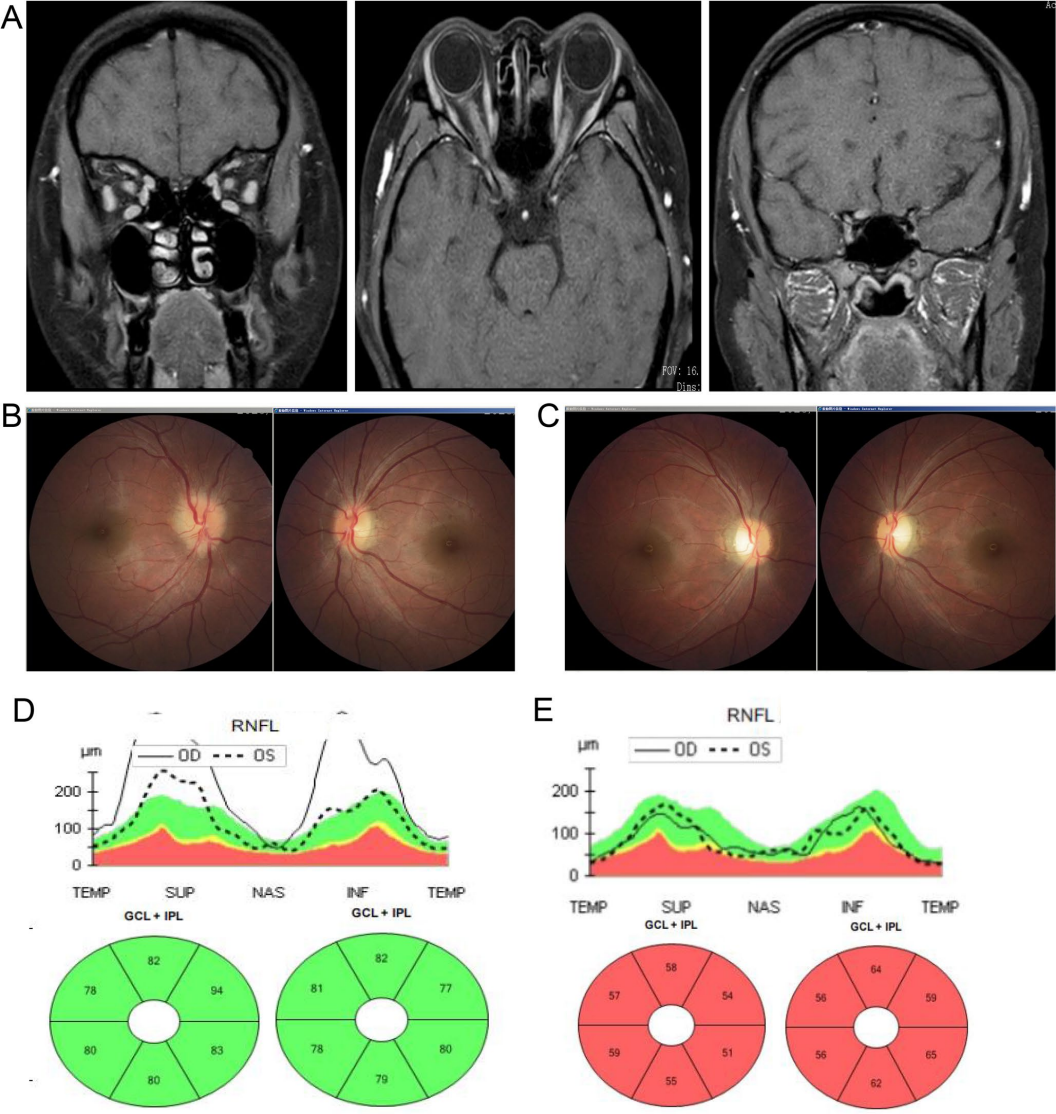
A 26-year-old woman without a significant past medical history presented with acute blurred vision of her both eyes with eye pain for 10 days during pregnancy (19 weeks). Thirteen days prior, she and her husband tested positive for coronavirus disease 2019 antigen after developing a fever, generalised body ache, and cough. The patient was serum myelin oligodendrocyte glycoprotein-immunoglobulin G-positive (1:32). (A). Optic nerve magnetic resonance imaging revealed the following: bilateral optic nerve orbital and canal segments, right optic nerve intracranial segment, and right chiasmal enhancement. (B). Bilateral optic disc oedema with Snellen decimal VA of HM/0.01 before treatment. (C) Bilateral optic disc pallor with Snellen decimal VA of 0.9/0.9 after 1.5 months. Optical coherence tomography revealed the following: a swollen retinal nerve fibre layer (RNFL) around the optic disc at initial presentation with normal ganglion cell layer (GCL) and inner plexiform layer (IPL) (D); 1.5 months after treatment, RNFL restored to normal with GCL and IPL thinning (E)
